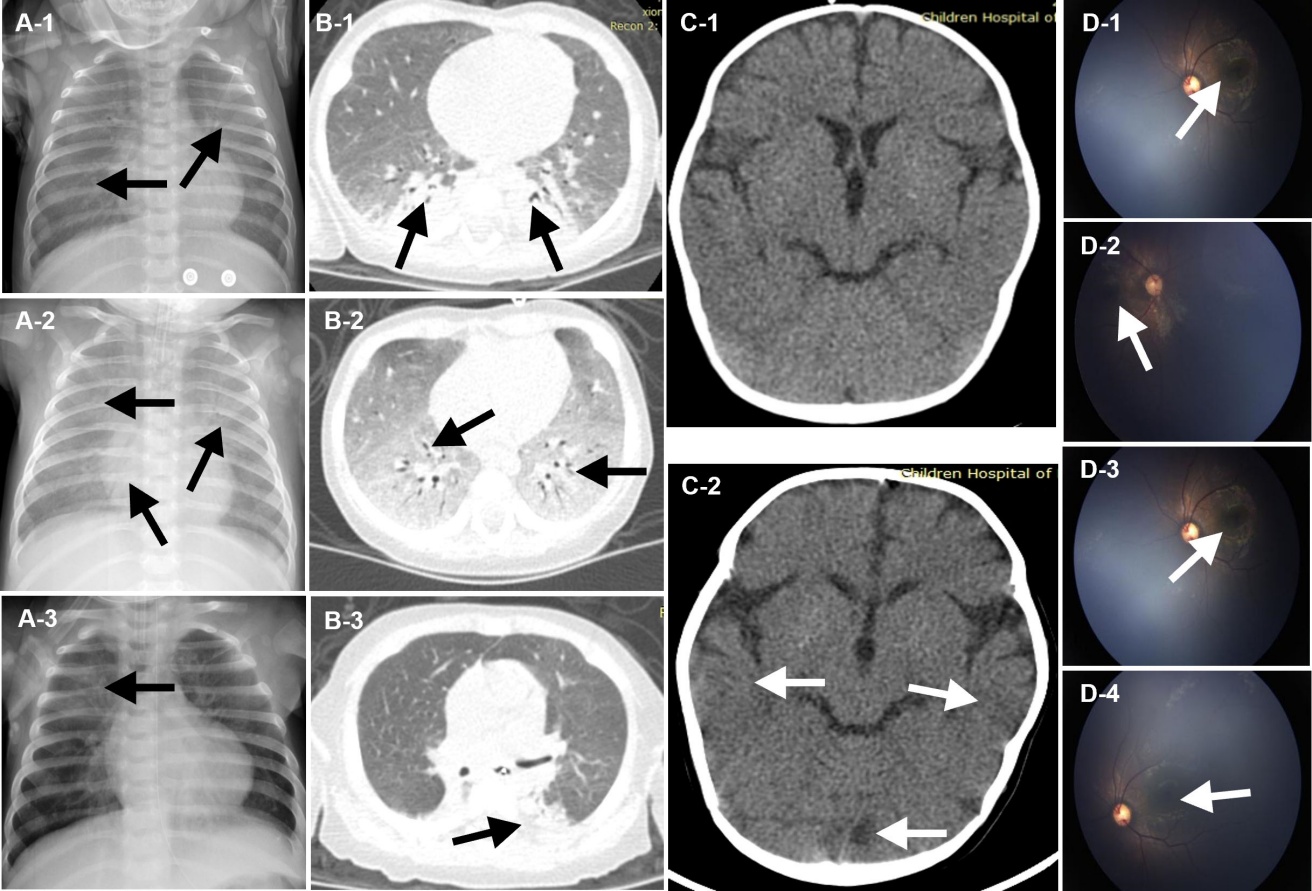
Chest Imaging, Neuroradiologic CT and Fundus perforator. The images shown in (A-1), (B-1), and (C-1) were acquired on admission to the emergency department of Children’s Hospital of Fudan University. Extensive exudation and parenchymal lesion of the chest was revealed (A-1, B-1, black arrows). And the image of the head was normal (C-1, white arrows). The image shown in (A-2), (B-2), and (C-2), (D-1) and (D-2) were acquired on day 14, after 10-day anti-microbial therapy. Progression of parenchymal lesion and interstitial infiltrates of the chest was revealed (A-2, B-2, black arrows), while multiple punctate calcifications of basal ganglia of the head was revealed (C-2, white arrows). Fundus examination suggested binocular leukoplakia lesions (D-1, D-2, white arrows). The image shown in (A-3) and (F) were acquired on day 36, after targeted anti-microbial for multiple pathogens. Lesions in lungs was nearly resolved (A-3, B-3, black arrows). The image shown in (D-3), (D-4) were acquired on day 72. Diffuse infiltrating lesions in his eyes and vascular white sheath were revealed (D-3, (D-4), white arrows).
